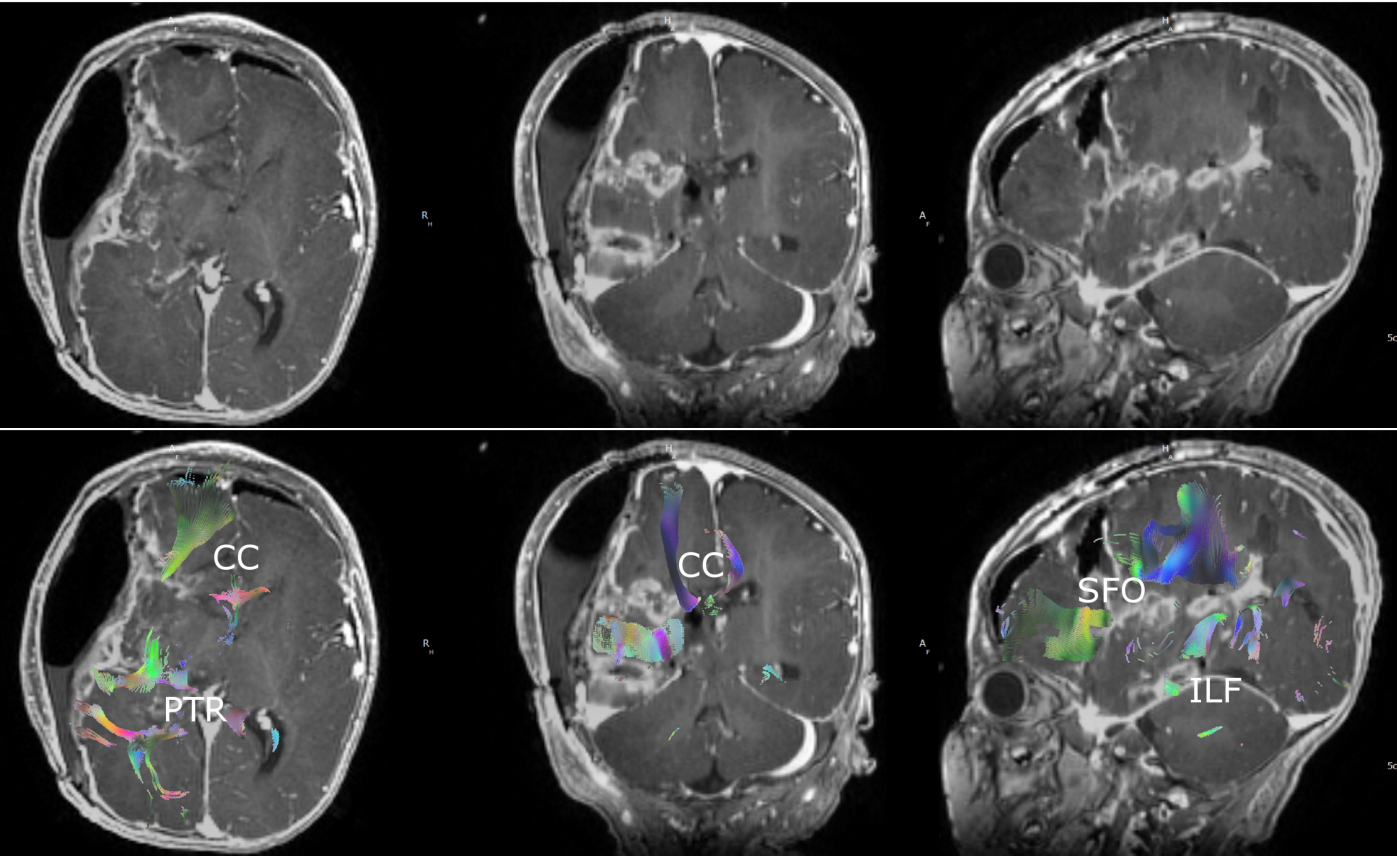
Postoperative magnetic resonance imaging (MRI) with diffusion tensor imaging Postoperative MRI demonstrating expected postoperative changes and manual seeding of right hemispheric white matter tracts demonstrating disconnections consistent with complete functional hemispherectomy. CC: corpus callosum; PTR: posterior thalamic radiations; SFO: superior fronto-occipital fasciculus; ILF: inferior longitudinal fasciculus
